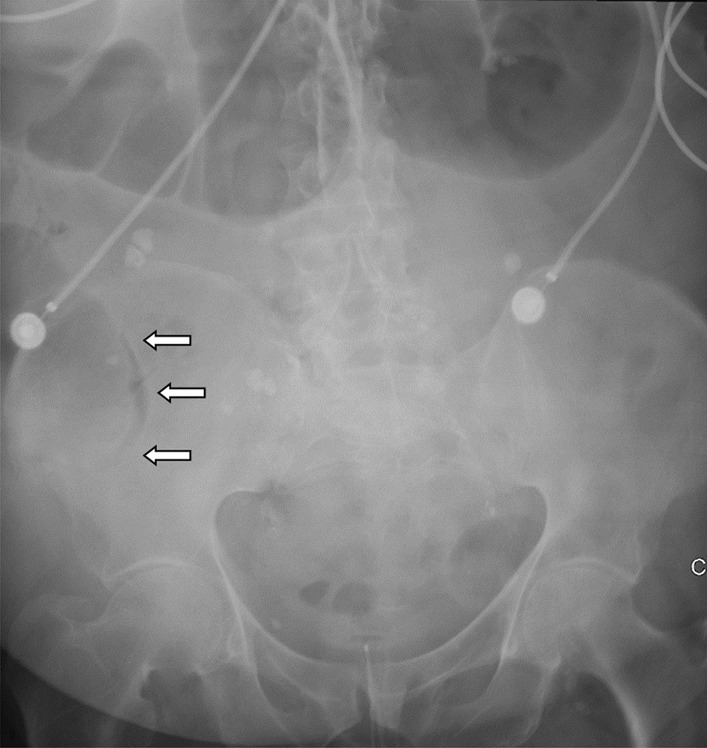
Abdominal X-ray showing pneumatosis intestinalis in the right colon (arrows)
